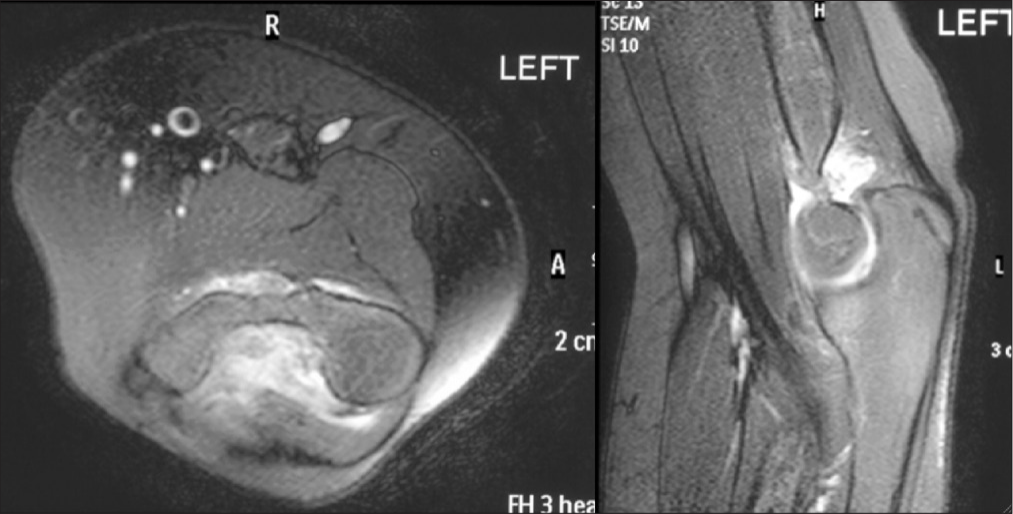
MRI scan of the left elbow showing joint effusion without evidence of bony injury. Loose bodies (osteochondral fragments) seen between trochlea and olecranon in saggital images, donor site was not identified. Ligaments, common flexor and extensor origins appeared intact
